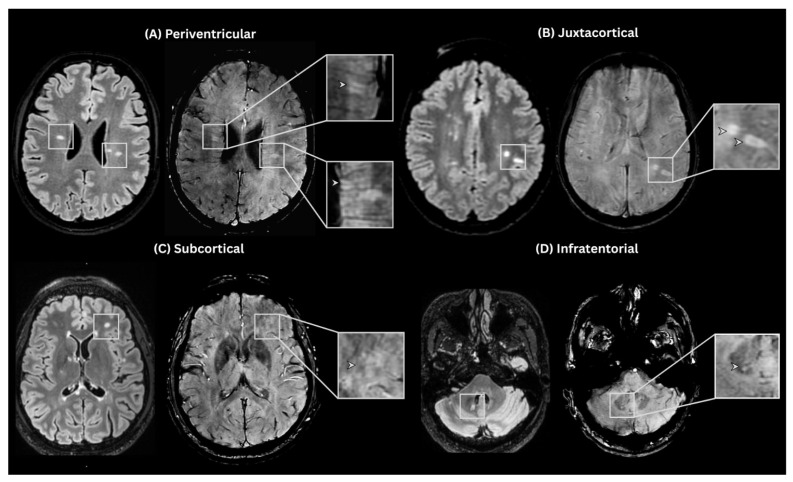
Examples of CVS-positive white matter lesions across four anatomical locations. Representative paired T2-FLAIR and SWAN images demonstrate CVS-positive lesions. On FLAIR, lesions appear as hyperintense foci, while the corresponding SWAN images reveal a central intralesional vein.
